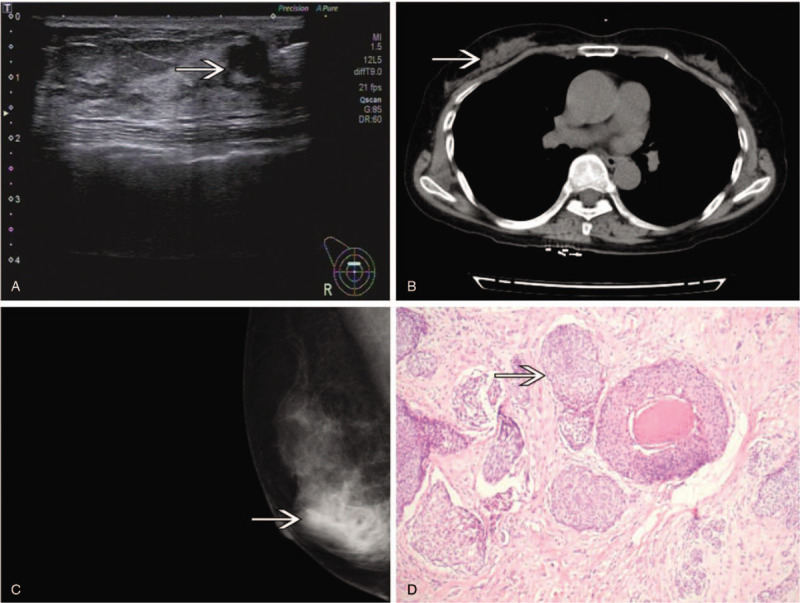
Ultrasound showed a regular, mixed echoic mass (A); CT scan (B) suggested an irregular mass (12 mm × 10 mm) behind the nipple (white arrow); Mammography (C) revealed a nodular high-density lesion (20 mm × 18 mm) with inhomogeneous; (D) revealed that ductal carcinoma in situ of the breast.
